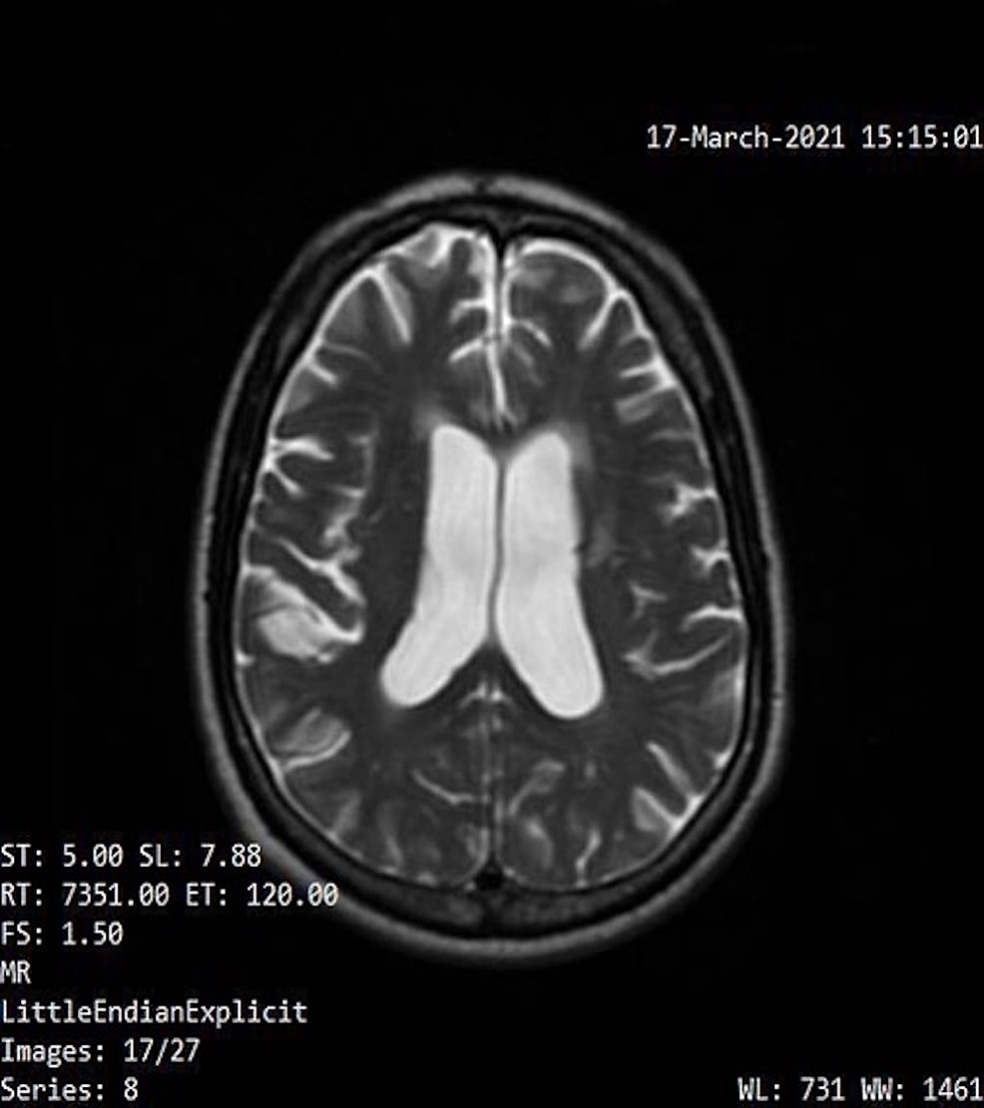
Patient MRI performed on March 17, 2021
